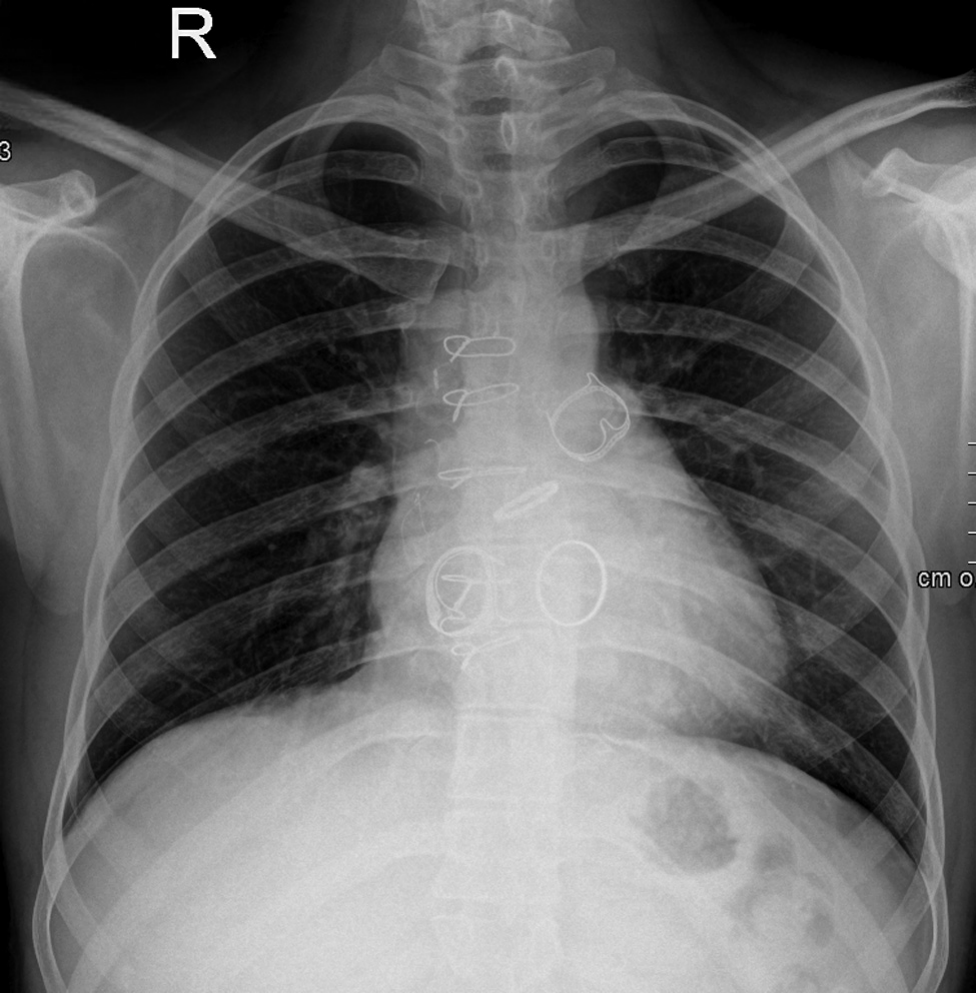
Post-surgery chest X-ray.
